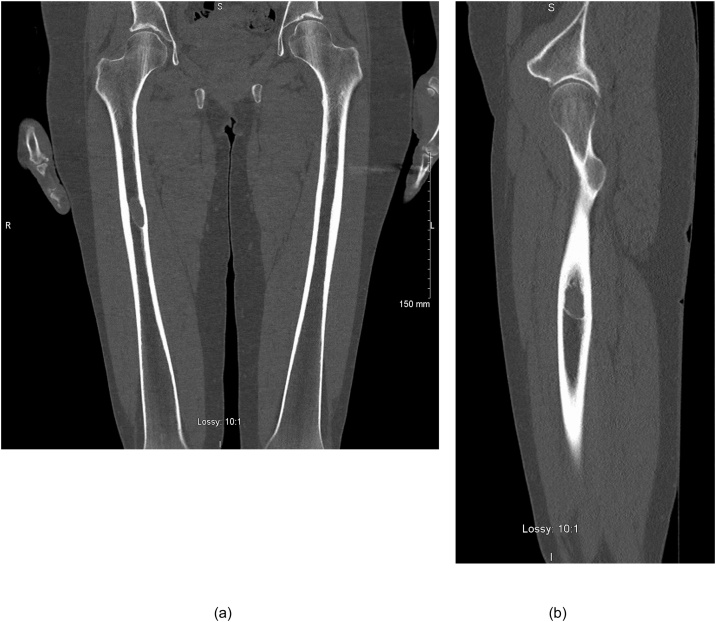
(a,b) Coronal and Saggital sections from CT Skeletal survey for paraproteinaemia*. Single 3.2 × 1.5 cm medium low attenuation lesion with a thin sclerotic margin in the midshaft of the right femur causing mild scalloping of the adjacent inner aspect of the medial cortex.
